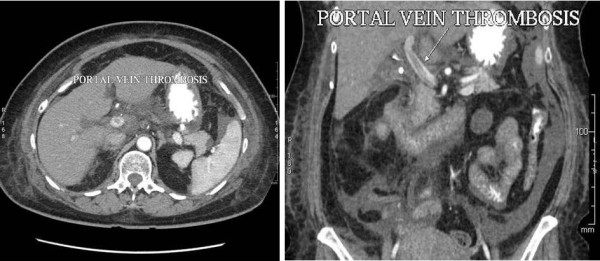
CT Abdomen showing thrombus in the portal vein (coronal and sagittal views).
